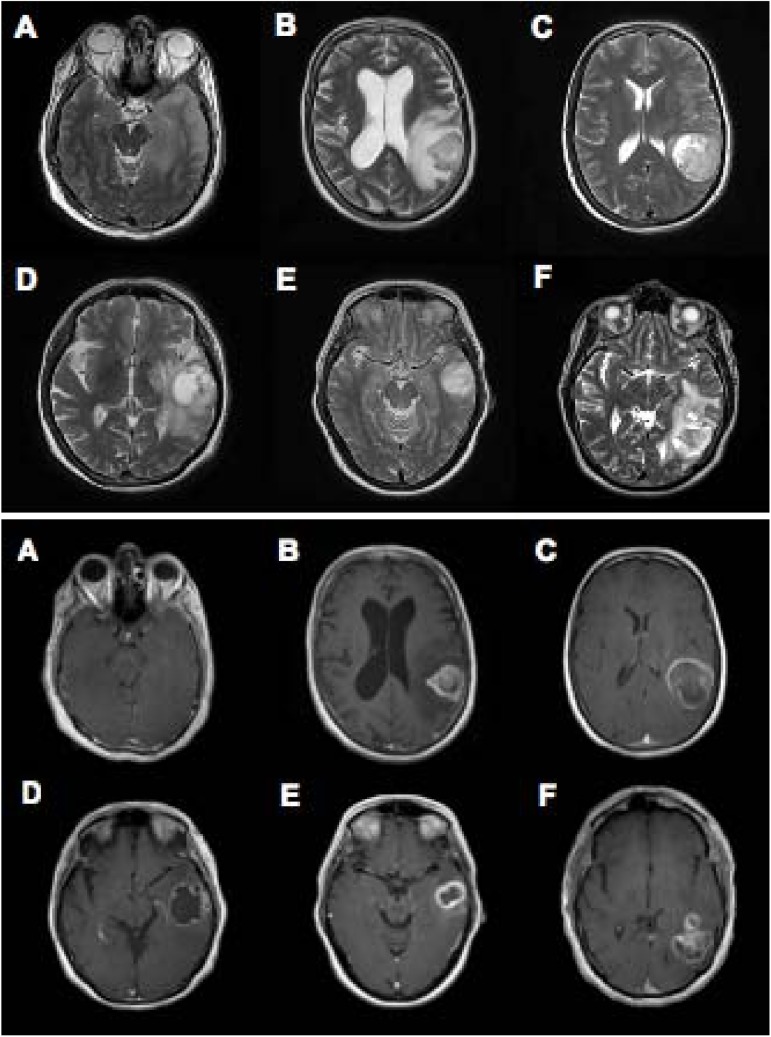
Preoperative MR Imaging. Representative axial slices demonstrating preoperative T2 weighted (upper panel) and contrast enhanced T1 weighted (lower panel) MR imaging demonstrating intracranial neoplasm in each patient.
